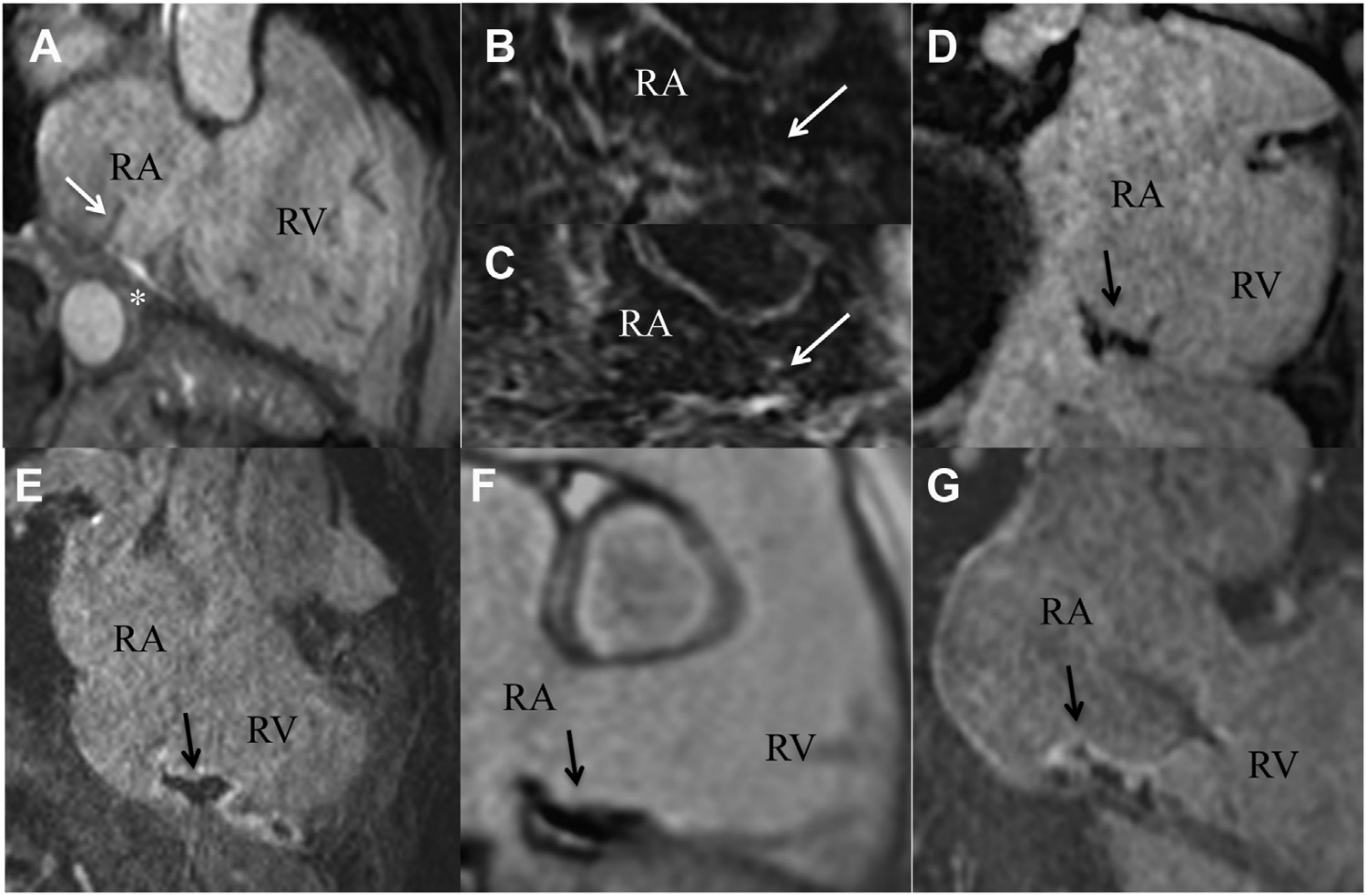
Acute lesion after radiofrequency ablation of the right cavotricuspid isthmus. A: Balanced steady-state free precision sequence image of the cavotricuspid isthmus (CTI) immediately after ablation. *White asterisk* indicates pericardial effusion. *White arrow* indicates a prominent eustachian valve. B, C: T2-weighted images preablation (B) and postablation (C) showing signal intensity enhancement of the isthmus line (*white arrows*). D: Noncontrast enhanced T1-weighted image of the CTI depicts acute necrotic lesions as signal intensity loss (*black arrow*). Inversion time was adjusted to suppress the acute lesion. E: Postcontrast early enhancement image shows hypoenhanced myocardium localized at the CTI, known as a microvascular obstruction as an acute ablation lesion sign. F: Phase-sensitive inversion recovery image depicts acute ablation lesion in terms of black, hypoenhanced myocardium. G: Postcontrast late gadolinium enhancement images led to partially enhanced radiofrequency ablation lesions (*white edge*) with black necrotic core (*black arrow*).
